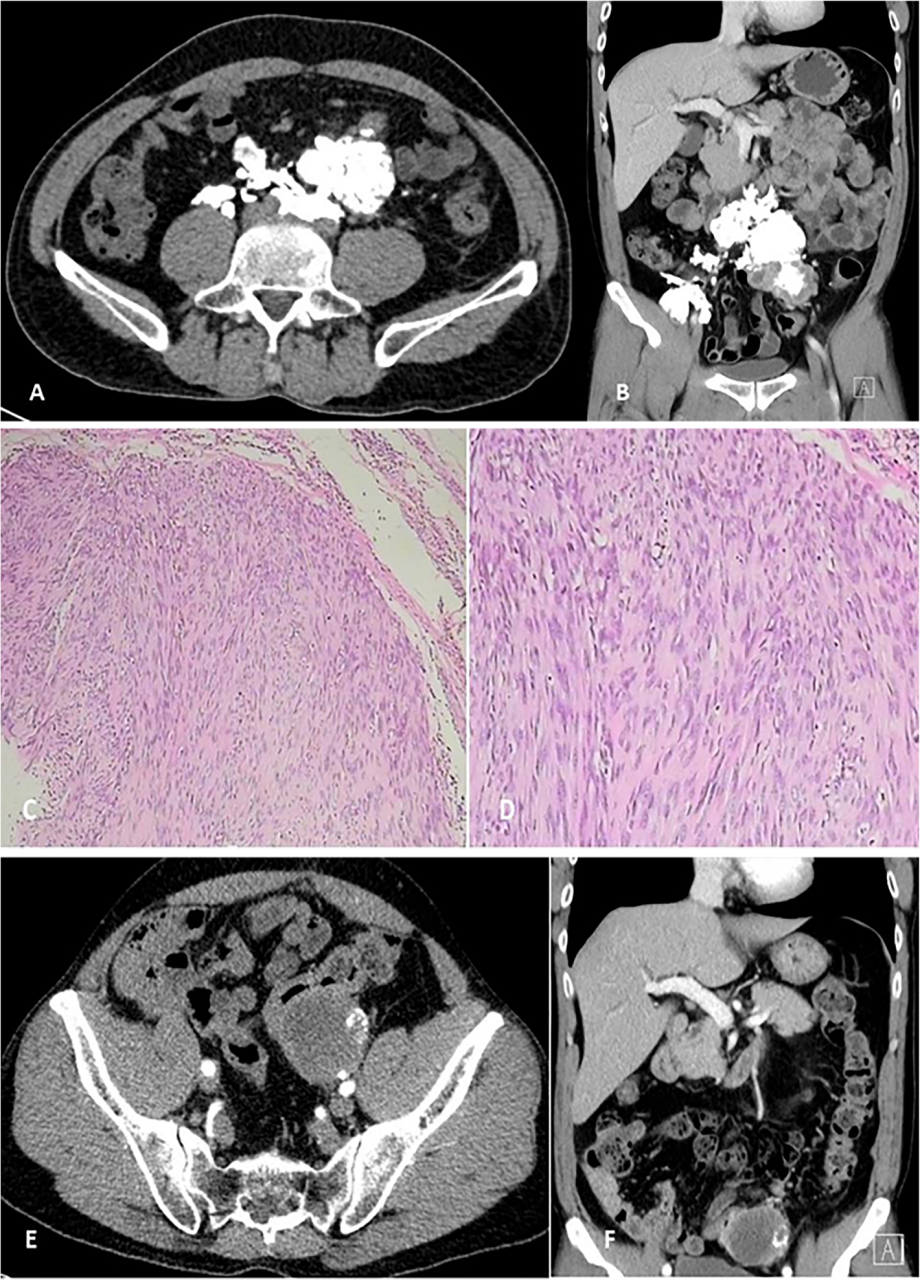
a and b preoperative CT: There were many irregular high and low mixed density shadows near the left lower abdomen and abdominal aorta, with clear demarcation and multiple patchy and banded calcification. The larger one was about 5.0 × 6.3. The enhanced lesions were unevenly enhanced. Pathology of (c and d) showed that spindle cell tumors were accompanied by a large number of hyaline degeneration and small focal ossification. e, f recurrence CT: There were a few masses, nodular high density lesions and soft tissue masses in the left lower abdomen. The largest size was about 4.8 *5.3 cm. On contrast-enhanced CT, uneven enhancement was seen, and nodular calcification was seen in them
